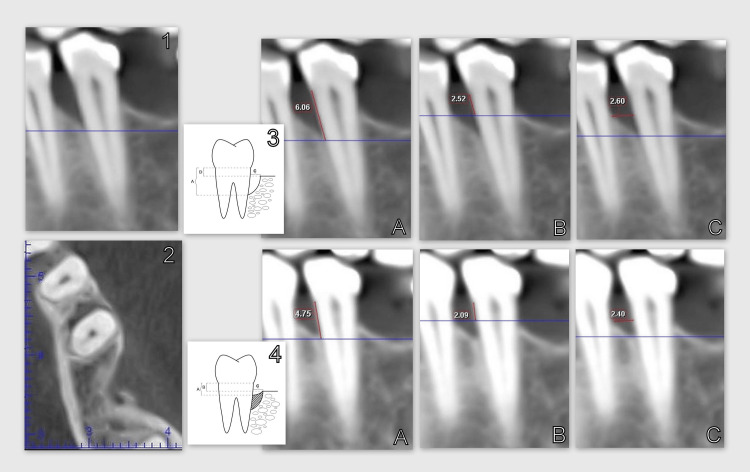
The CBCT images before and after regenerative therapy with PRP 1: Coronal plane of the vertical defect on CBCT; 2: Transversal plane of the vertical defect on CBCT; 3: CBCT parameters studied before regenerative therapy; 3A: Parameter A (zero months); 3B: Parameter B (zero months); 3C: Parameter C (zero months); 4: CBCT parameters studied six months after regenerative therapy; 4A: Parameter A (six months); 4B: Parameter B (six months); 4C: Parameter C (six months) CBCT: Cone beam computed tomography
